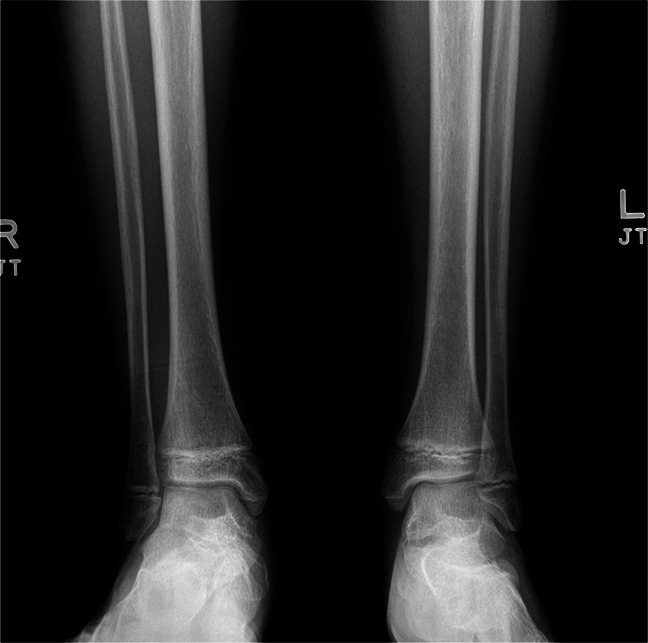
Radiograph showing an AP standing view of bilateral ankles at the 6-month postoperative visit demonstrating an open distal tibia physis on the right.
